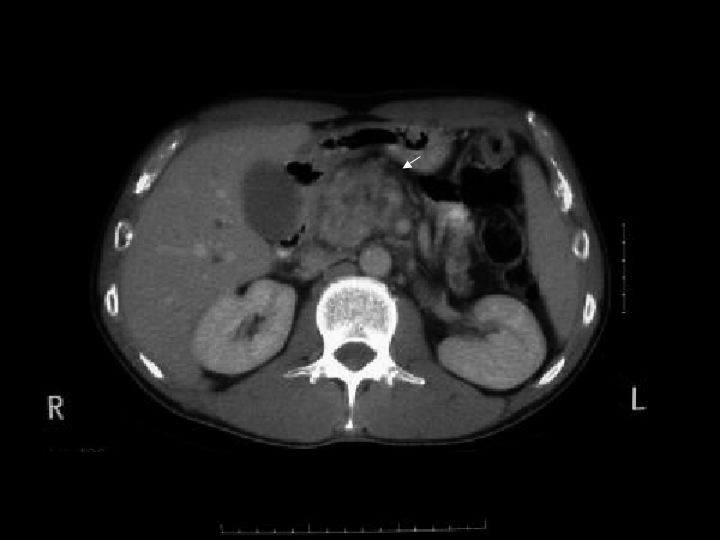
Computed tomography scan demonstrating mass at the head of the pancreas.
